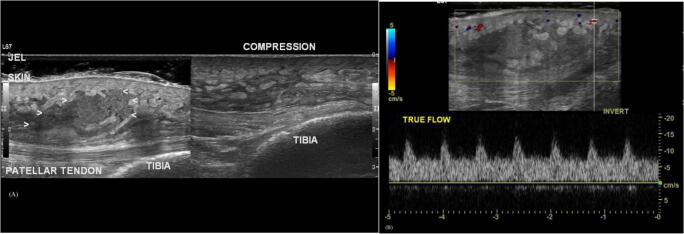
a There is a compressible subcutaneous collection in the infrapatellar anterior knee. There are mobile echoes in it. b Doppler shows that there is local hypervascularity. Case courtesy of Dr Maulik S Patel, Radiopaedia.org, rID: 50551
